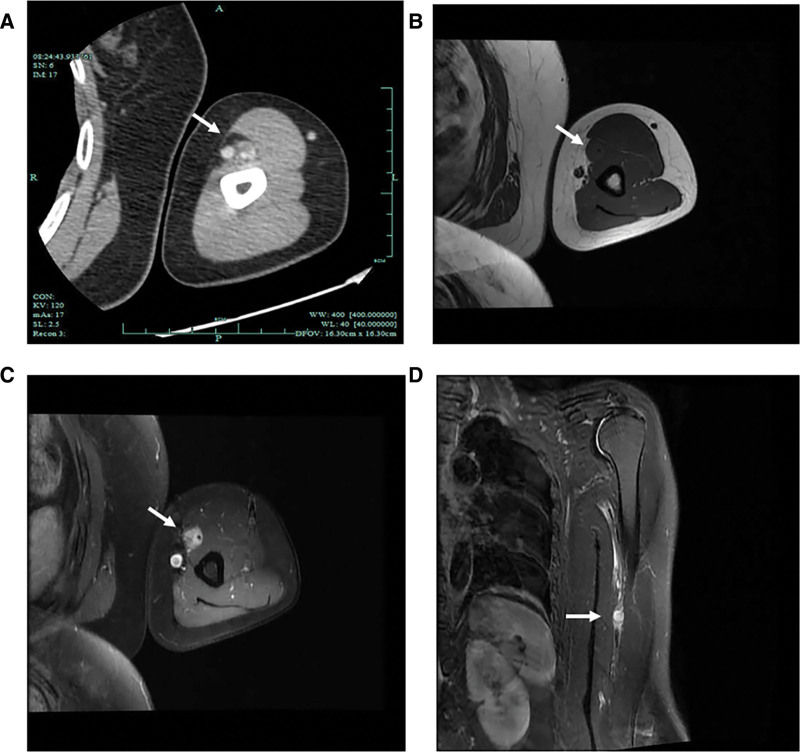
Computed tomography (CT) and magnetic resonance imaging (MRI) of the tumor. (A) Contrast-enhanced CT of the left upper limb shows nodular soft tissue density shadow inside the left upper arm with significantly enhancement, and the demarcation between the lesion and surrounding soft tissue is clear, closely adjacent to the BA (white arrow). (B–D) MRI demonstrates the lesion is moderate intensity but significantly enhanced on T1-weighted imaging and has high intensity in T2 fat suppressed sequence (white arrows).
